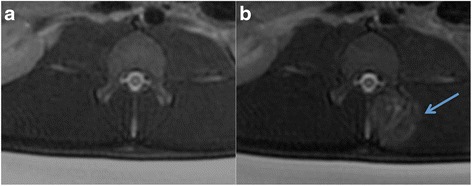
Axial T2-weighted images. a Acquired before FUS. b After FUS treatment. The lesion is clearly visible as hyperintense signal, suggesting the presence of edema. The sonication energy used for the depicted joint was 800 J. Smaller lesion sizes were generated with smaller sonication energies
